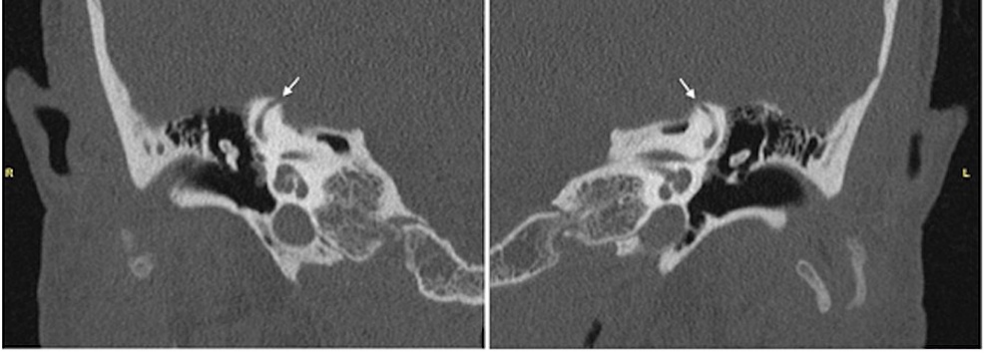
A 53-year-old woman presenting with migraines, vertigo, dizziness, and right-side greater than left-side tinnitus, hyperacusis, and chewing internal sound amplification High-resolution CT scans demonstrate area of 5 mm and 4 mm dehiscence of the (R) right and (L) left superior semicircular canal apices, respectively. Citation from Chung et al. [10] CT, computed tomography
